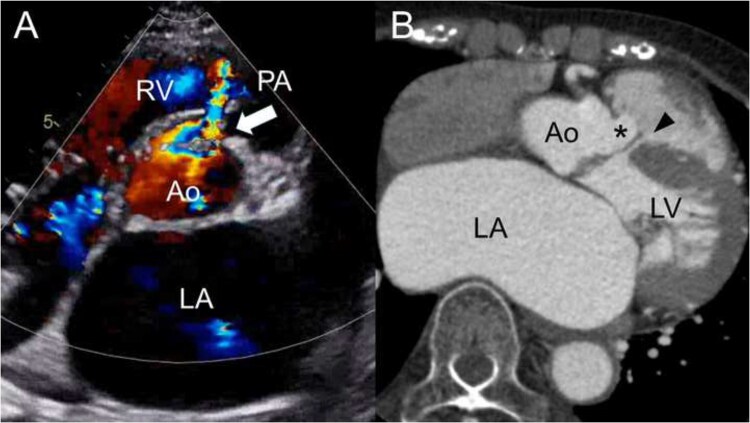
Preoperative Image of Ventricular Septal Defect. (A) Transthoracic echocardiography shows a ventricular septal defect (arrow) with a left-to-right shunt. (B) Computed tomography angiography shows right sinus of Valsalva aneurysm (asterisk) herniating into the restrictive ventricular septal defect (arrowhead) beneath the right coronary cusp, with prolapse into the left ventricle. Abbreviations: Ao = aorta; LA = left atrium; LV = left ventricle; PA = pulmonary artery; RV = right ventricle.
